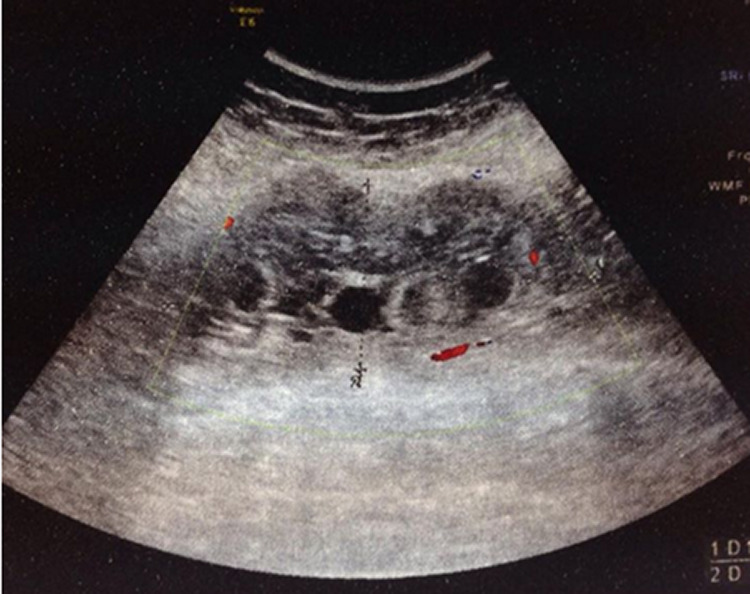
Superficial Doppler ultrasound in the transversal plane (A) and longitudinal plane (B) of the thigh shows an ill-defined multilocular cystic mass of the middle one-third of the posterior compartment of the thigh measuring 13 cm × 5.5 cm and containing an echogenic peripheral portion that is finely vascularized on color Doppler.
